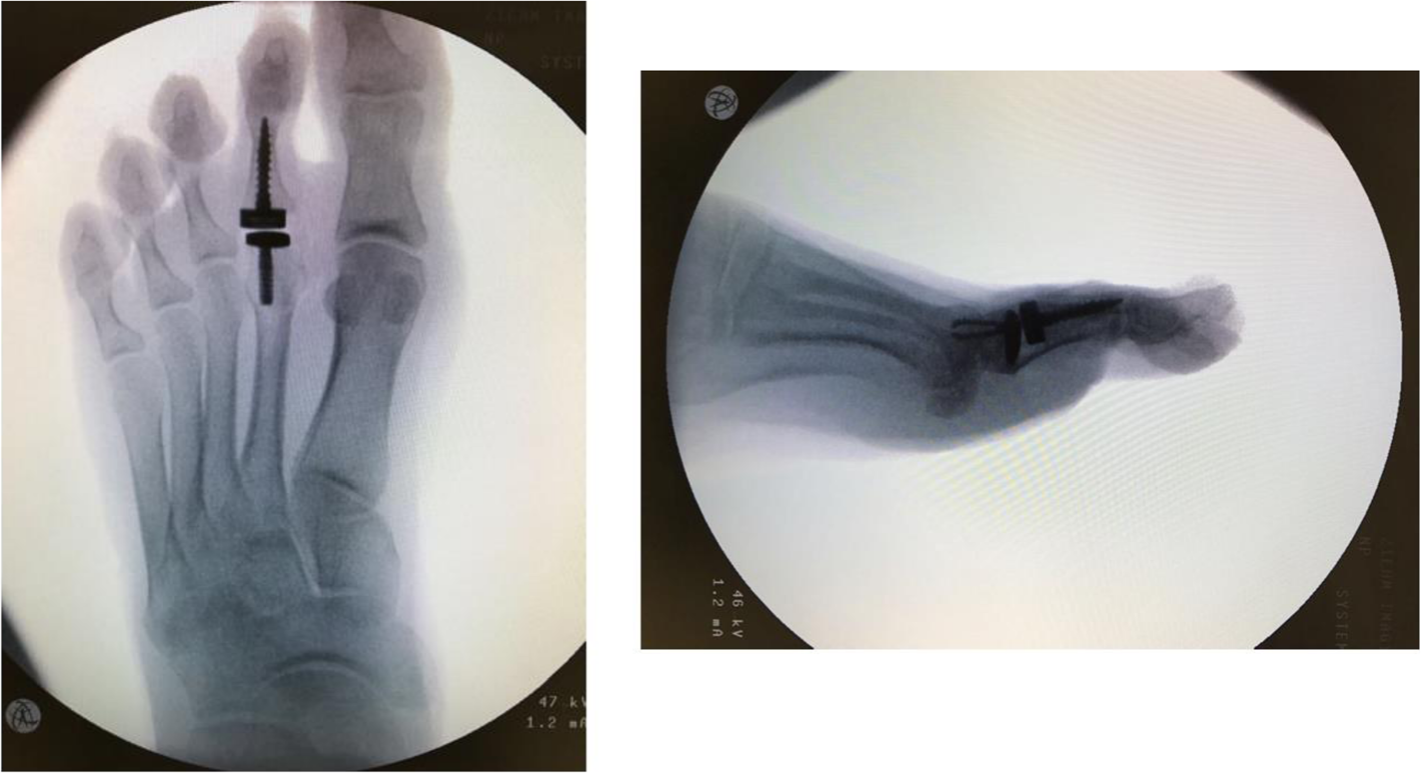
Radiographic appearance of the implant (antero-posterior and lateral views)
